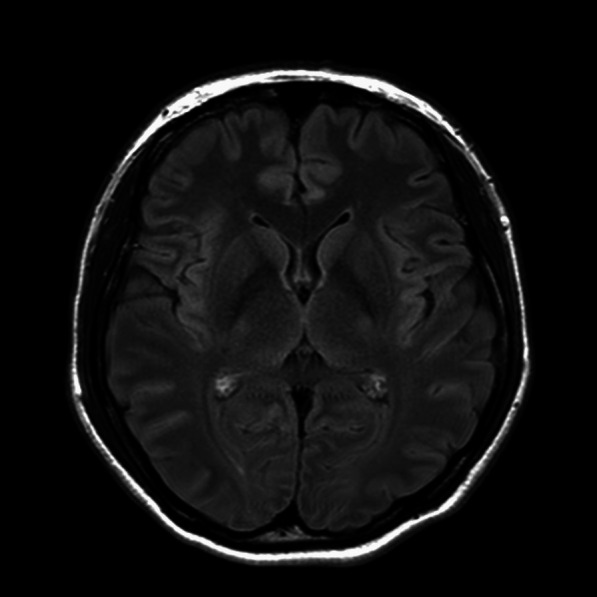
Sulcal hyperintensity on post-contrast FLAIR implying blood–brain barrier breakdown due to vasoconstriction and contrast exposure
